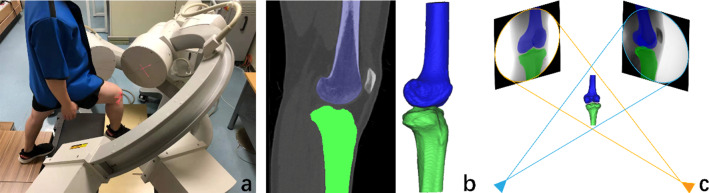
a Dual fluoroscopy was used to record the motion of the subject's right knee while he performed a lunge motion. b CT imaging was used to create 3D models of both the femur and tibia. c The 3D models were synchronized with the dual fluoroscopic images to reproduce the motion of the subject's knee during the movements
